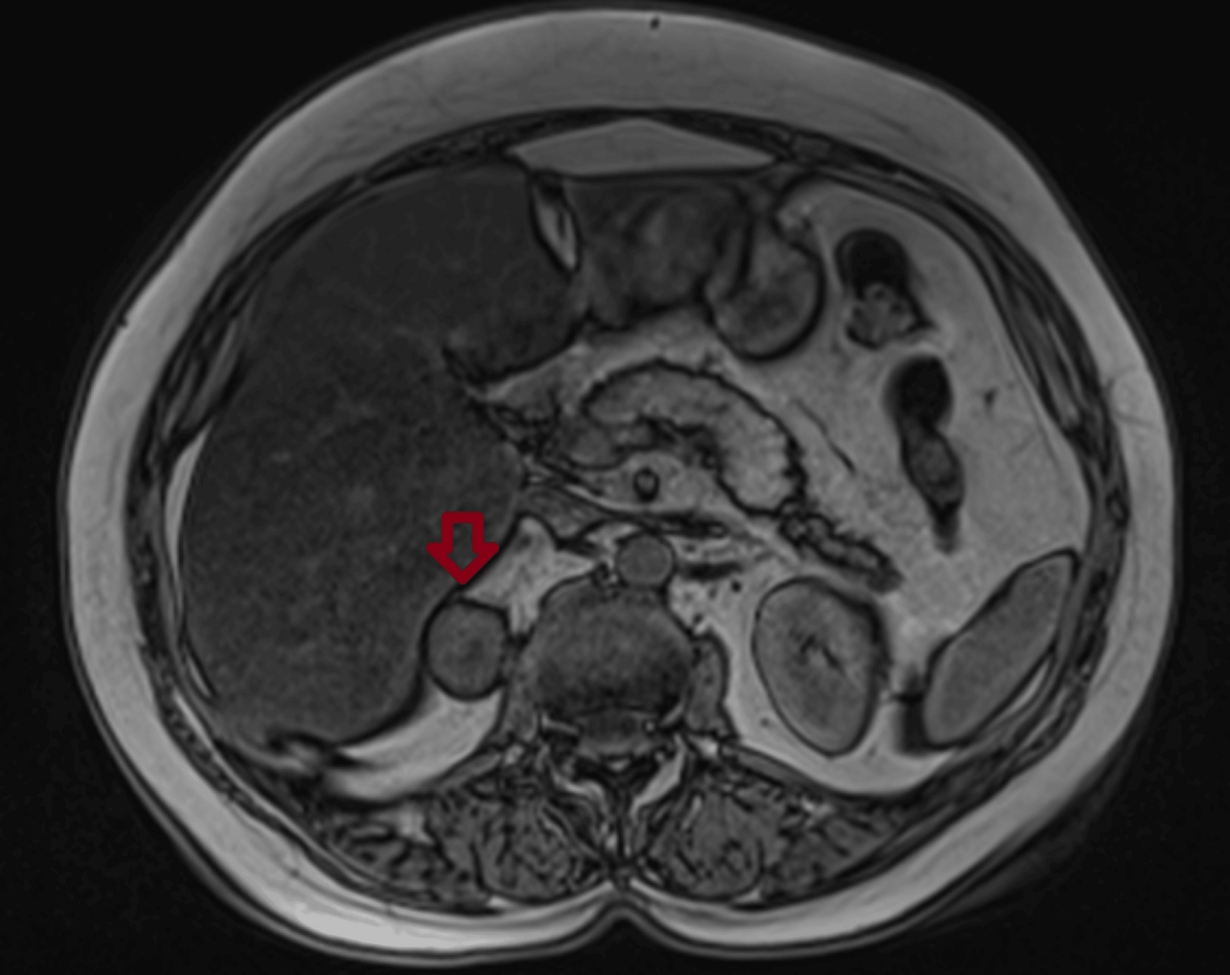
Adrenal imaging findings of Patient 4 An abdominal MRI (transverse plane) of a 57-year-old woman with a right adrenal incidentaloma measuring 30 mm.
